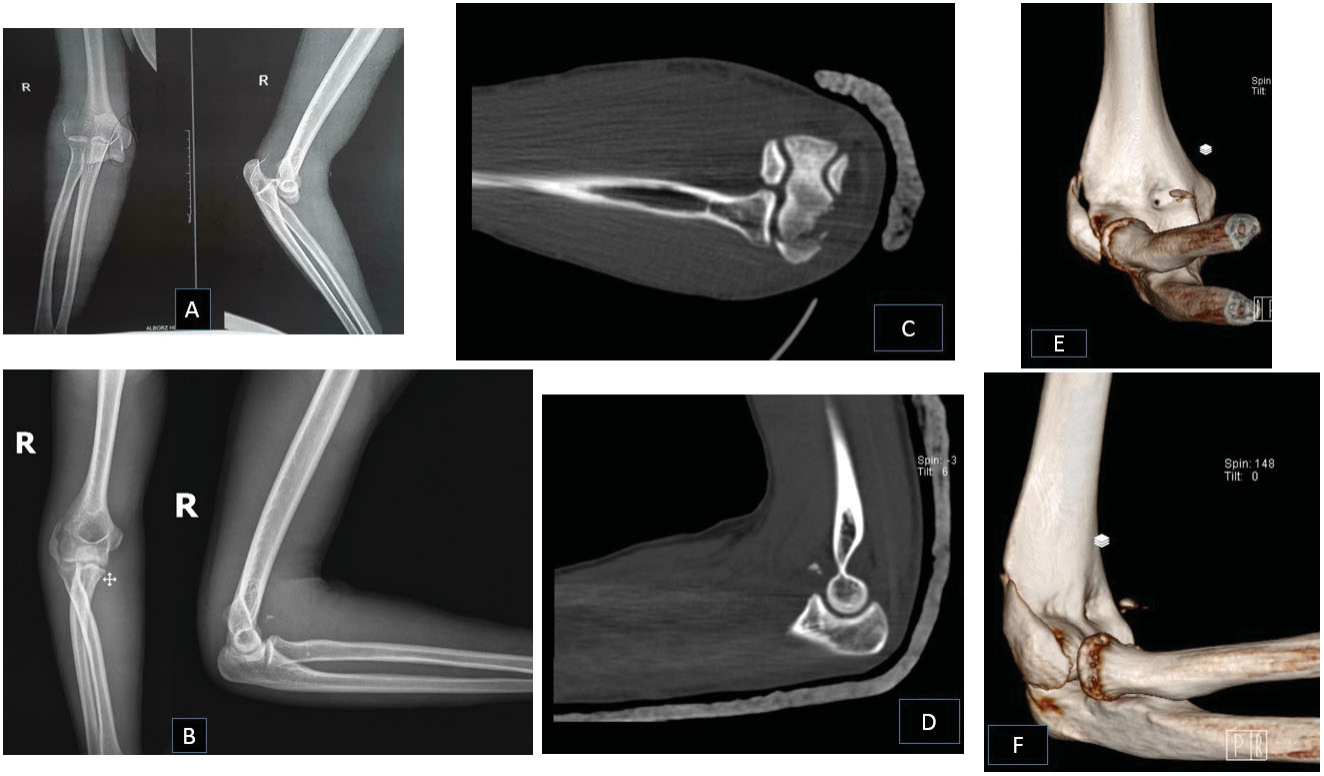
The primary radiographs of the patient before (A) and after (B) close reduction. Axial (C), sagittal (D) and 3D CT scan (E&F). Note the coronoid fracture and extension of condyle fracture to the radiocapitellar joint.
